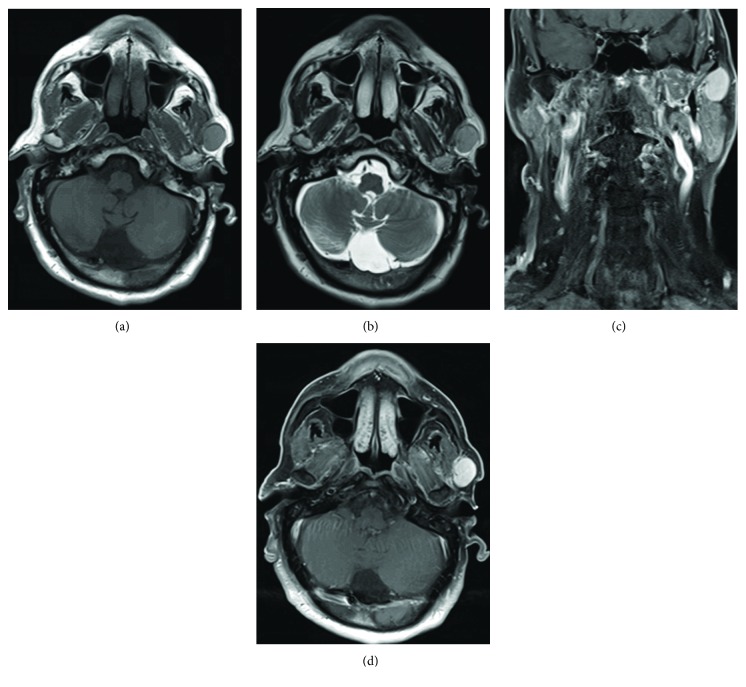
(a–d) MRI neck. (a, b) The mass at the left parotid space with slightly high signal intensity to the muscles on both axial T1 and T2 spin echo MR weighted images. (c, d) Coronal and axial postcontrast axial fat suppressed T1 MR weighted images demonstrate avid homogenous enhancement of the tumour.
